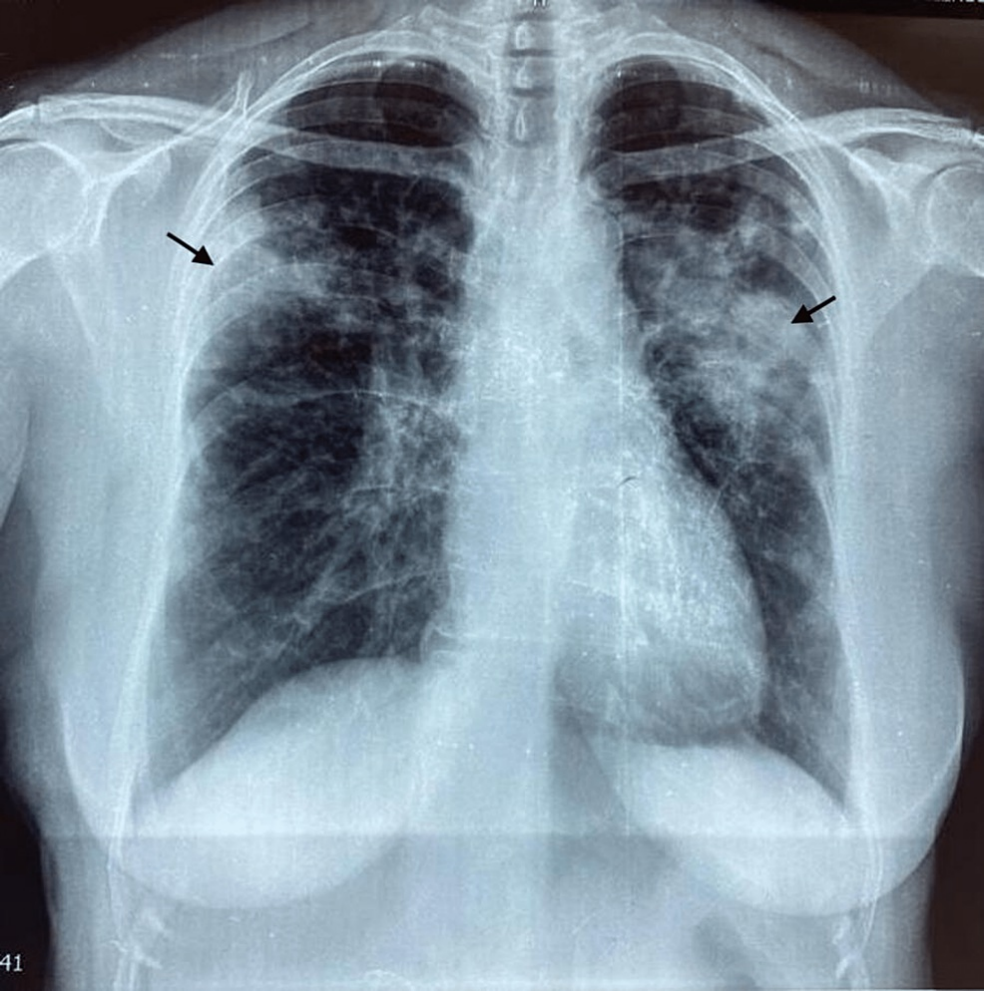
Posteroanterior chest radiograph demonstrates bilateral alveolar syndrome with foci condensation. The image shows homogeneous dense opacity, poorly limited left upper, a dense opacity located in the subpleural level of the right upper lobe extended bilaterally, and opacities of nodular type in the right.
